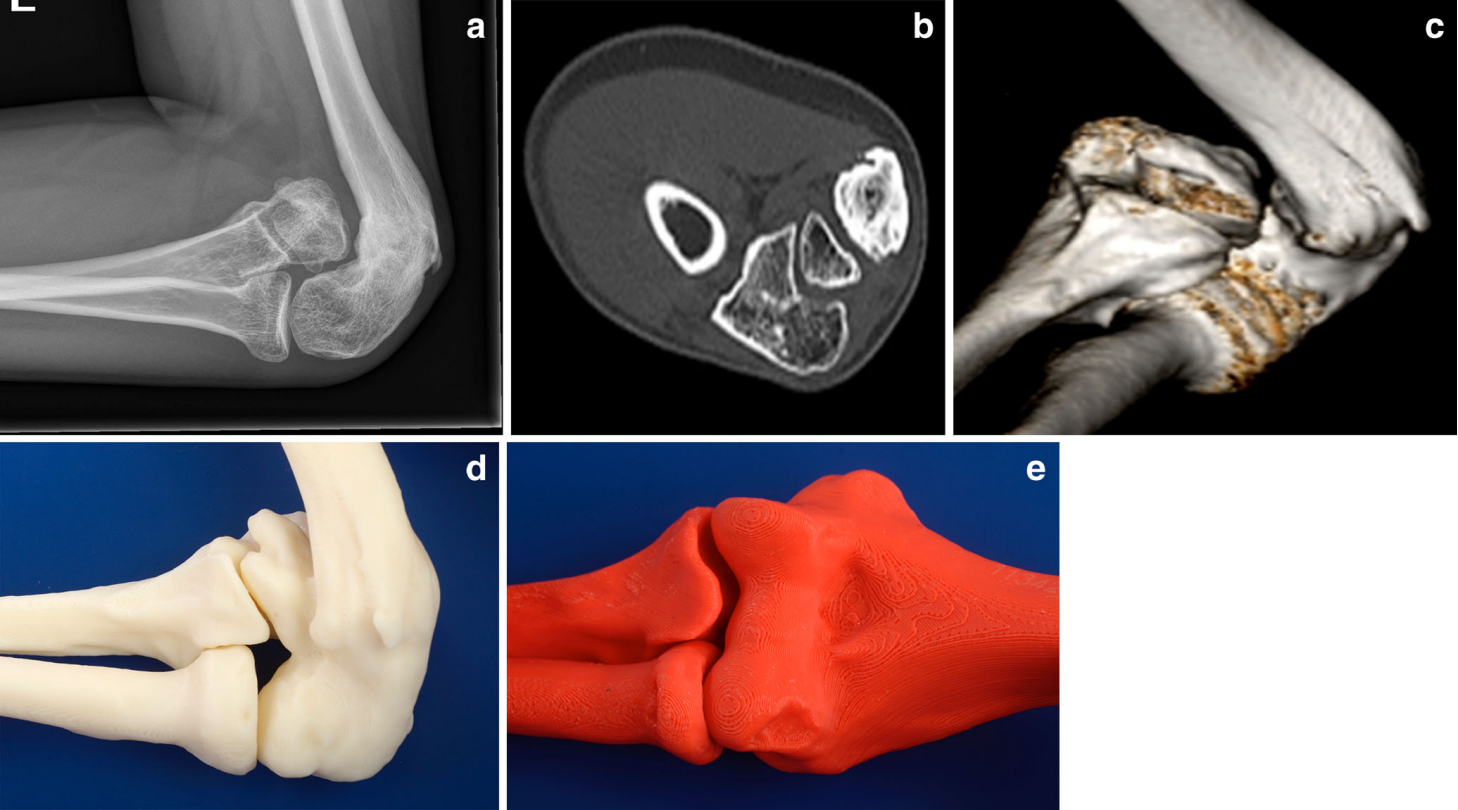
For complex cases, a 3D CT and 3D models of the affected and the mirror-imaged healthy elbow will provide better insight in the nonunion and associated deformity. This patient had a war-related injury to his elbow as a child and presented 11 years later. Plain radiographs suggested an elbow dislocation as seen on the lateral radiograph (a). 2D CT imaging (b) and 3D-reformatted CT imaging (c) showed a malunion of the distal humerus with associated medial condyle nonunion. In addition, there was overgrowth of the radial head and capitellum. The proximal radio-ulnar joint, the radio-capitellar joint, and the relation between the proximal ulna and medial condyle nonunion are intact. 3D-printed models of the affected (d) and mirror-imaged healthy side (*red*) (e) provide valuable insight.
